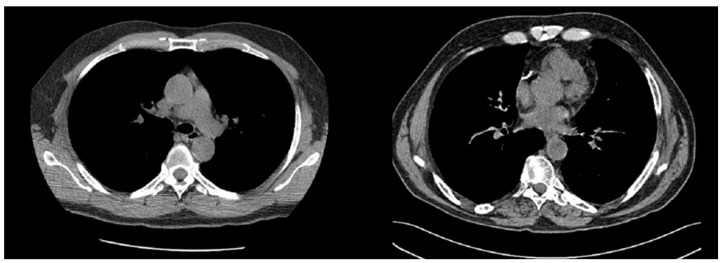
CT slice DICOM images [24].
